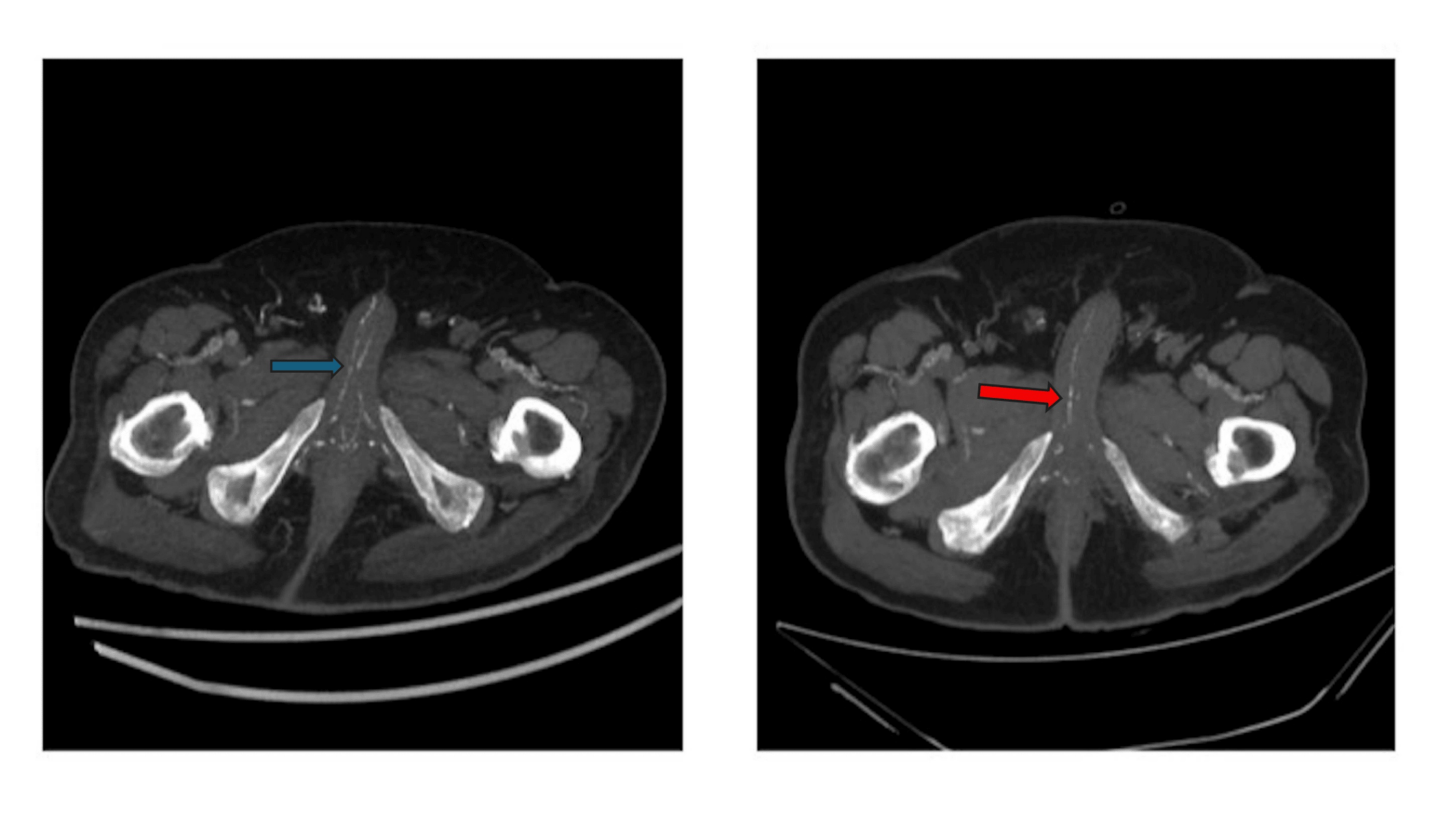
The axial maximum intensity projection reformatted computed tomography (CT) images reveal a significant interval worsening of calcifications in the penile arteries. The image on the left shows more pronounced calcifications in both the cavernosal and dorsal arteries of the penis (blue arrows), indicating disease progression when compared to the baseline examination on the right for the same arteries (red arrows).
